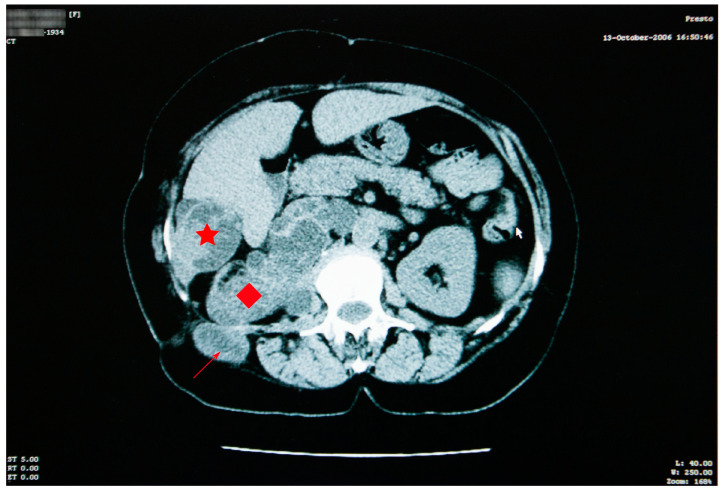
Abdominal CT scan showing multiple cysts, confluent in the right kidney space (red diamond), invading the retroperitoneal area, in the sixth right liver segment (star) and in the right iliac fossa and right paravertebral area (arrow).
